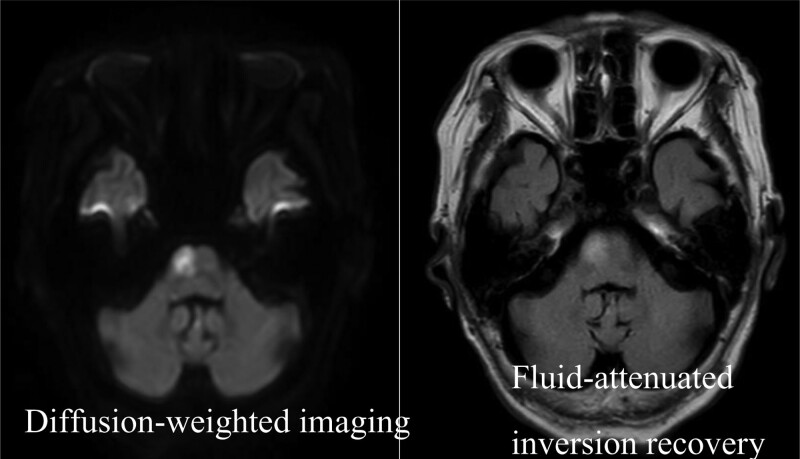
Brain magnetic resonance imaging on hospitalization d 8. Brain magnetic resonance imaging shows cerebral infarction in the pyramidal tract from the pons to the medulla oblongata.
